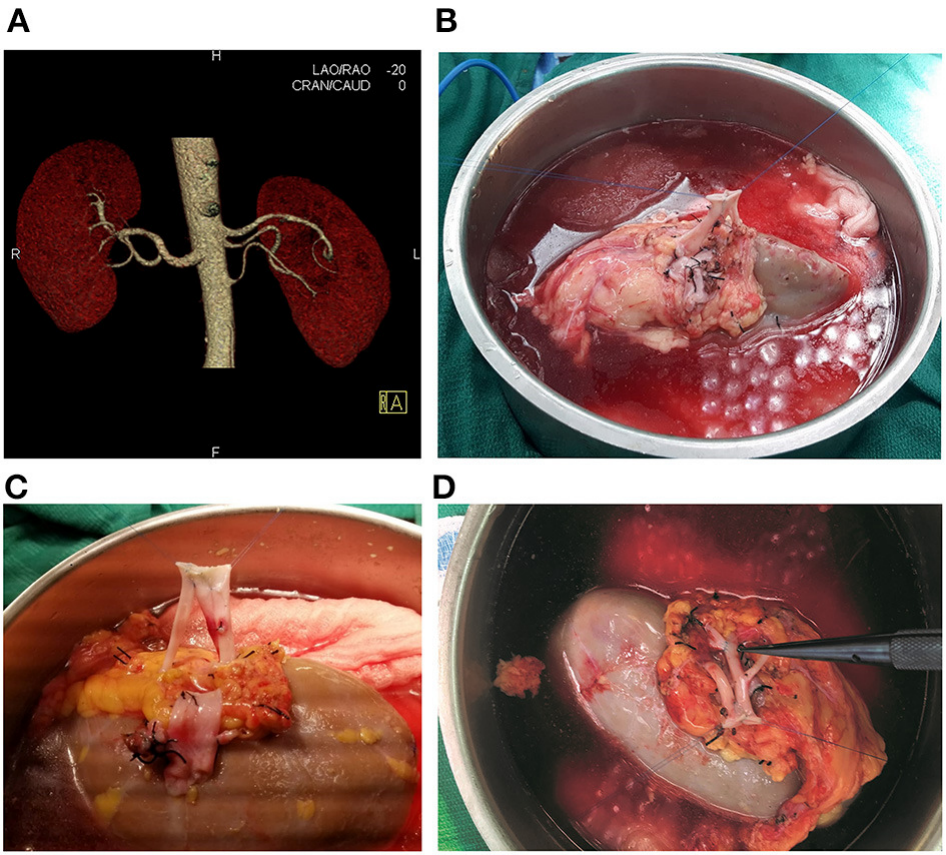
(A) Computed Tomography Angiography showing the left kidney with three renal arteries. (B) Living donor kidney with two arteries, conjoined side-to side with 8-0 Prolene. (C) Living donor kidney with three arteries, with all three renal arteries conjoined side-to side with 8-0 Prolene. (D) Living donor kidney with two main renal arteries and an upper pole accessory renal artery. The two renal arteries were anastomosed side-to-side with 7-0 Prolene, and the accessory upper pole artery was anastomosed end-to-side into one of the main renal arteries with 8-0 Prolene suture inside the renal hilum.
